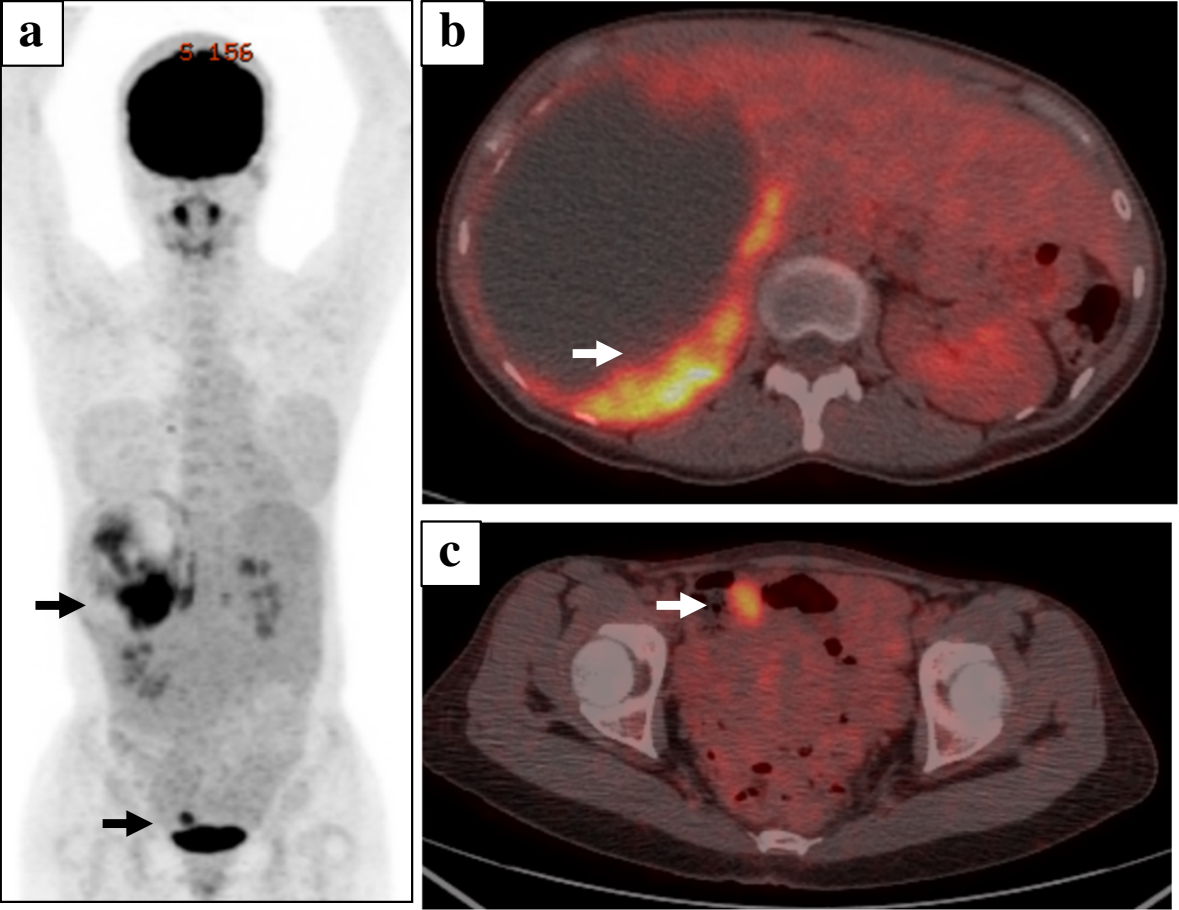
Findings of FDG-PET. a The fusion image of FDG-PET shows abnormal accumulation in the liver and lower abdomen (*black arrows*). b Cross-section of the upper abdomen indicates abnormal accumulation in the mural nodule in the liver (*white arrow*). c Cross-section of the lower abdomen indicates abnormal accumulation of the omental tumor (*white arrow*)
